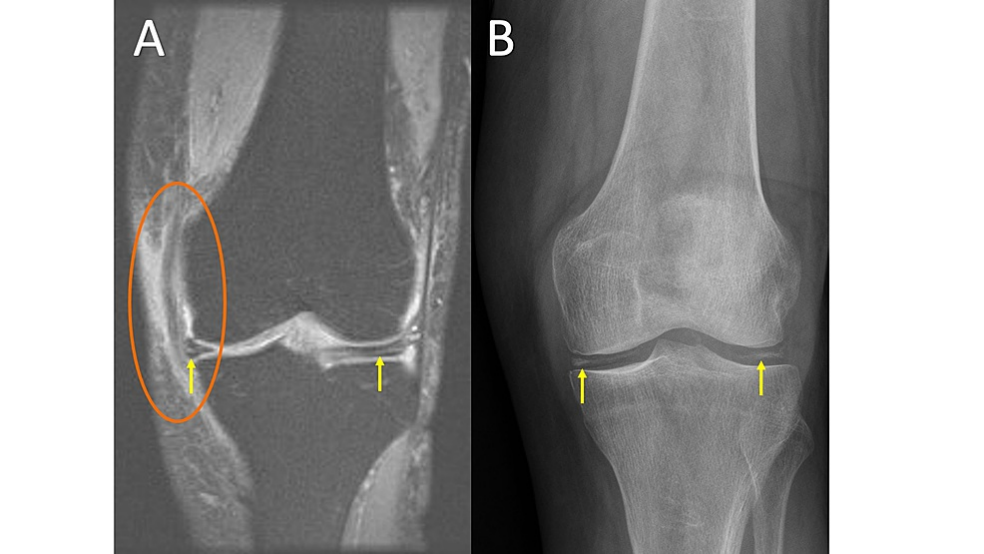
Calcium Pyrophosphate Deposition Disease MRI coronal T2 fat saturation (FS) (5A) demonstrates a linear hyperintense T2 signal within the menisci (arrows). Incidentally, the medial collateral ligament is thickened and heterogenous with increased T2 signal both within and around the ligament consistent with a sprain (circle). Anteroposterior (AP) knee radiograph (5B) demonstrates chondrocalcinosis of the menisci (arrows).
